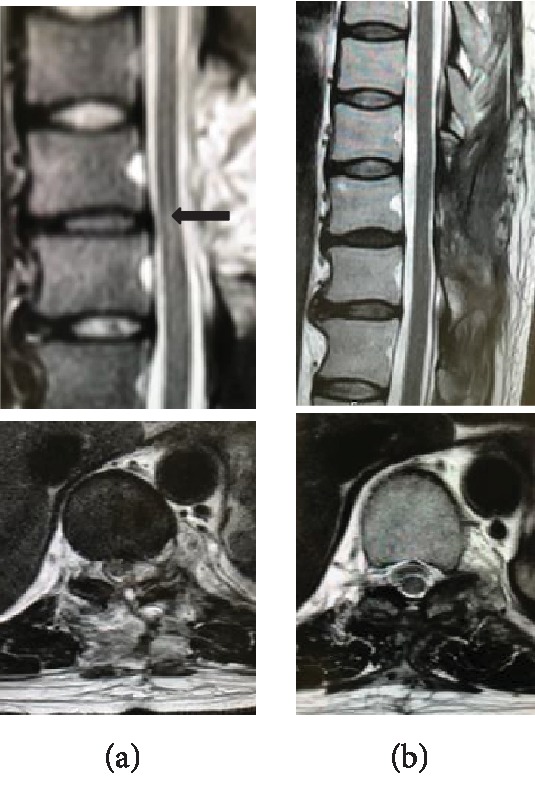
MRI taken on the 7th day after surgery (a) showed decompression of the spine and recorded a high signal in the spinal cord, indicating local ischemic changes of the spine at the Th 10 level ((a) arrow). The MRI taken 3 months after the surgery (b) showed no ischemic change or the facet effusion.
